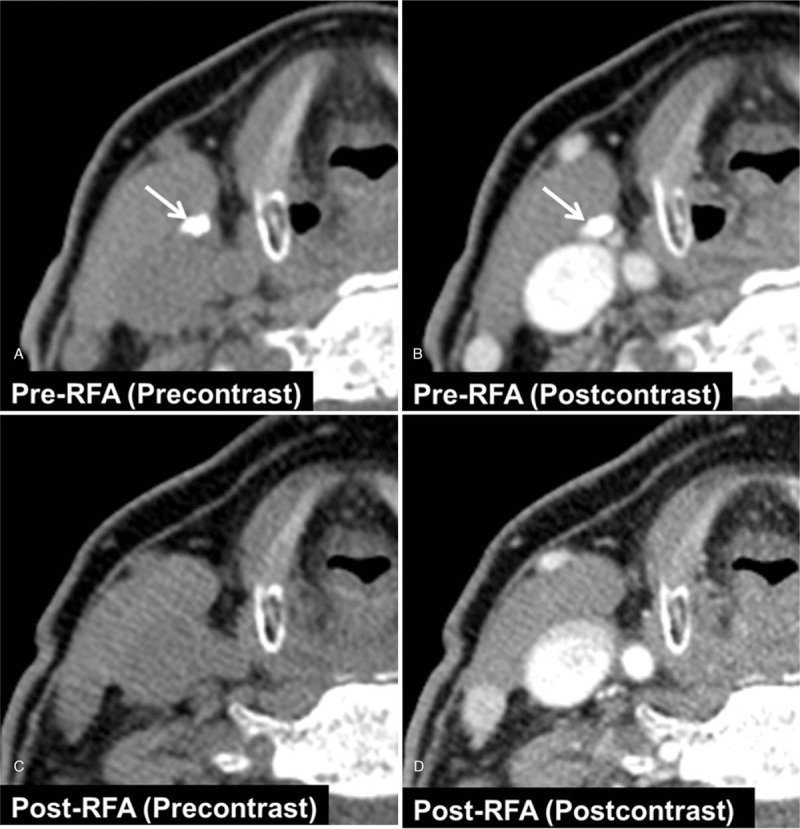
Pre- and post-RFA thyroid CT. (A, B) Pre-RFA CT shows a suspicious lymph node with dense macrocalcification at the right level III (arrow). (C, D) Follow-up thyroid CT performed 3 years after RFA shows no demonstrable residual lesion.
